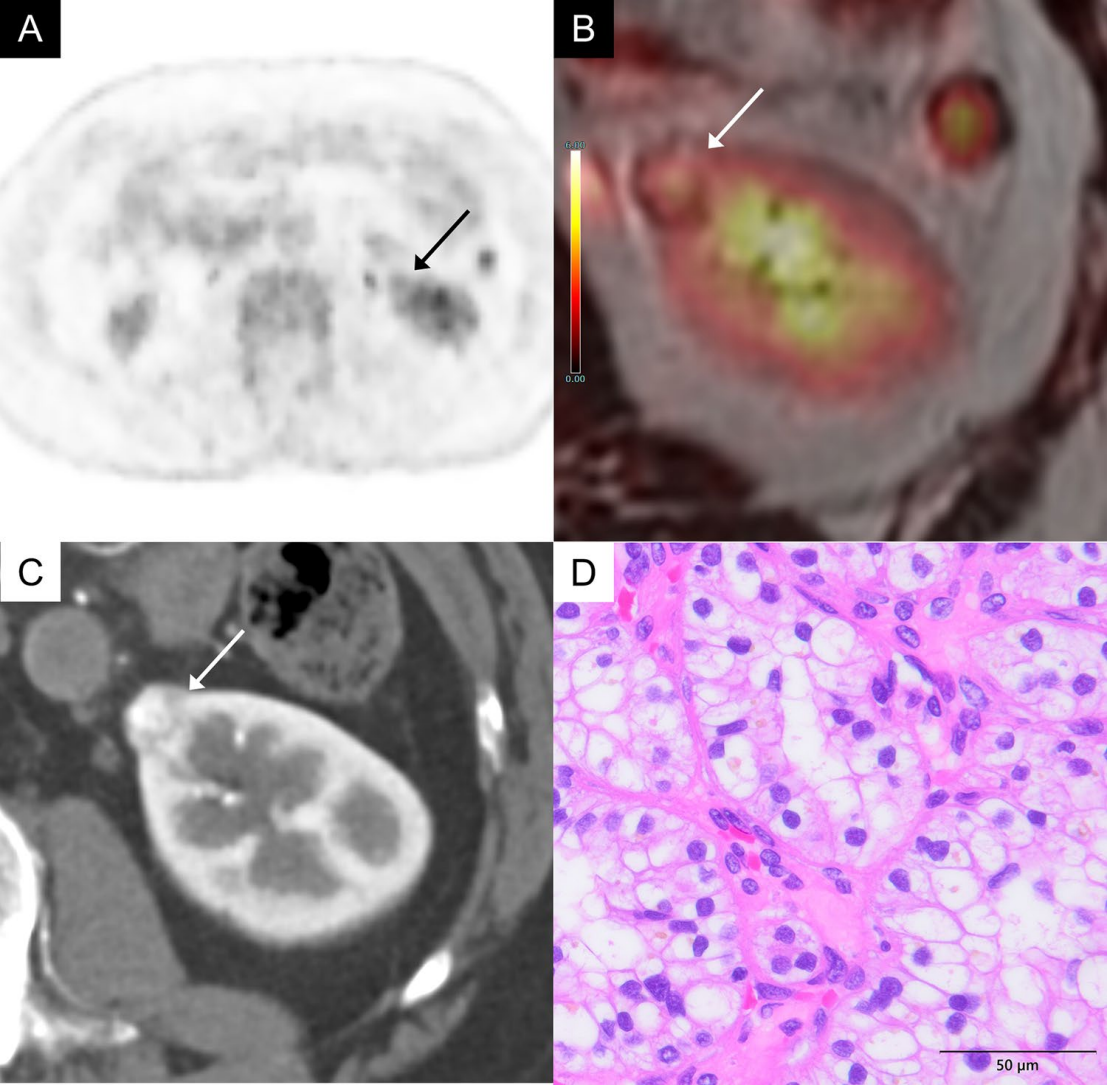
A 60-year-old man with a clear cell renal cell carcinoma (World Health Organizaion/International Society of Urological Pathology (WHO/ISUP) grade 2) of the left kidney. A In the FDG-PET image (grayscale), the uptake of the left renal tumor (arrow) is almost the same as that of the renal cortex, making tumor identification difficult. B Axial FDG-PET/magnetic resonance imaging (MRI) image shows mild FDG uptake in a mass of the left kidney (arrow), showing a maximum standardized uptake value (SUVmax) 2.8, while normal renal cortex shows an SUVmax 2.3. C Axial contrast-enhanced computed tomography (CT) in the corticomedullary phase shows a heterogeneously intense enhancement in the mass (arrow). D The tumor cells have predominantly clear cytoplasm. Conspicuous nucleoli are visible only at a high-power view (× 400)
